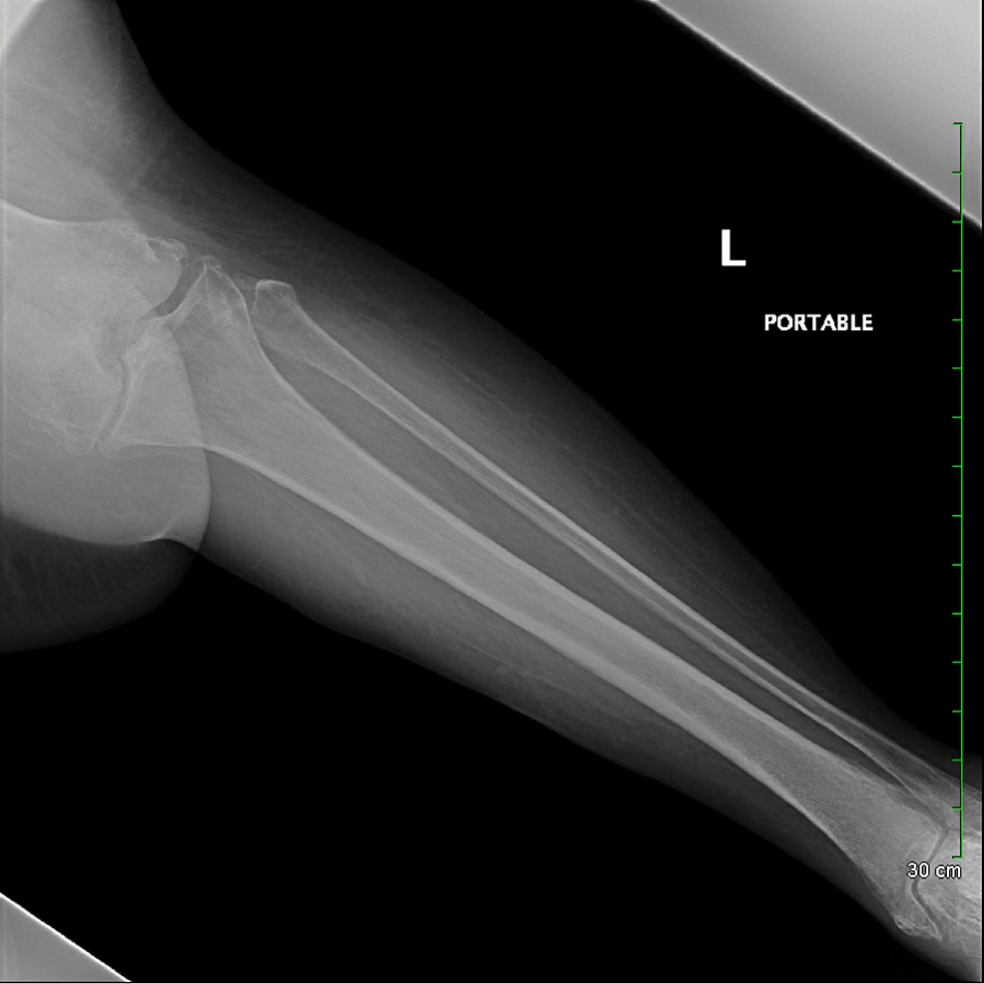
An X-ray of the right tibia and fibula, frontal and lateral views. The bones are demineralized, which is a limiting factor for nondisplaced fracture evaluation. No dominant acute fracture in this long bone study.
